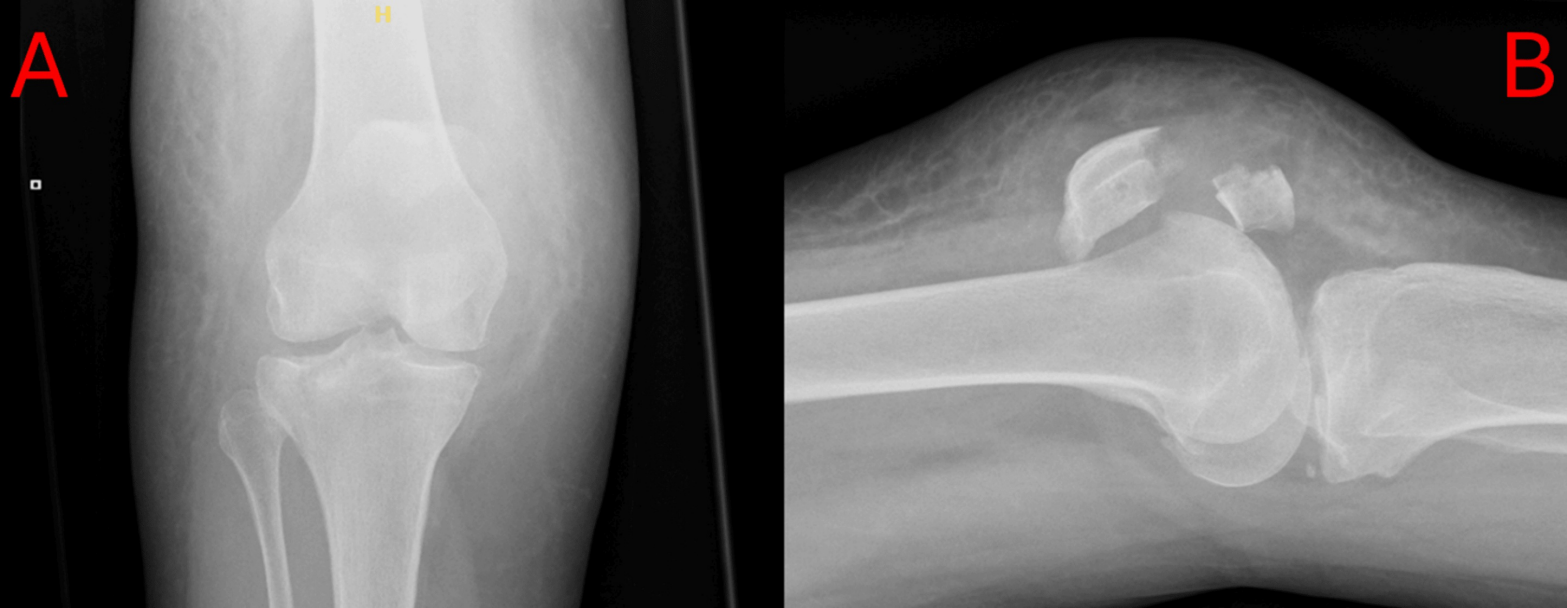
Preoperative anterior to posterior (a) and lateral (b) X-rays of the injury A) Plain anterior to posterior radiograph of the patient's right knee. This image shows a Schatzker 2 [1] lateral condylar fracture; B) Plain lateral radiograph of the patient's right knee. This image shows a displaced, midbody patellar fracture with associated haematoma.
